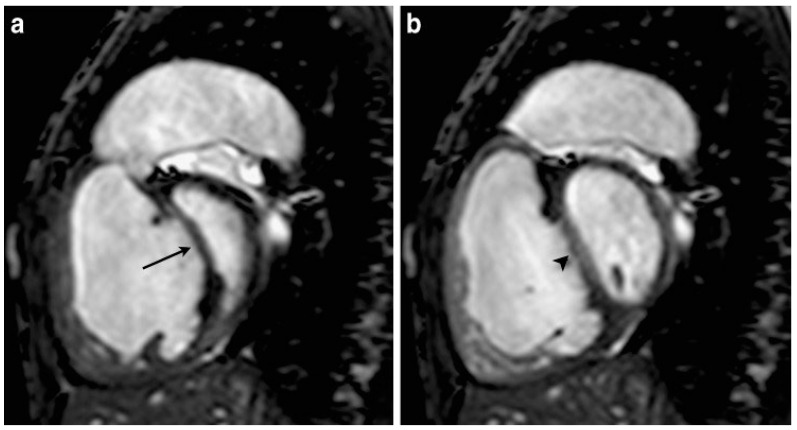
An 18-year-old man with severe idiopathic PH. Short-axis steady-state free precession cine MR image at early diastole (a) and end diastole (b). Arrow showing septal shift toward left ventricle causing a D-shape left ventricle. Reproduced from Ref. [23] under CC BY license.
